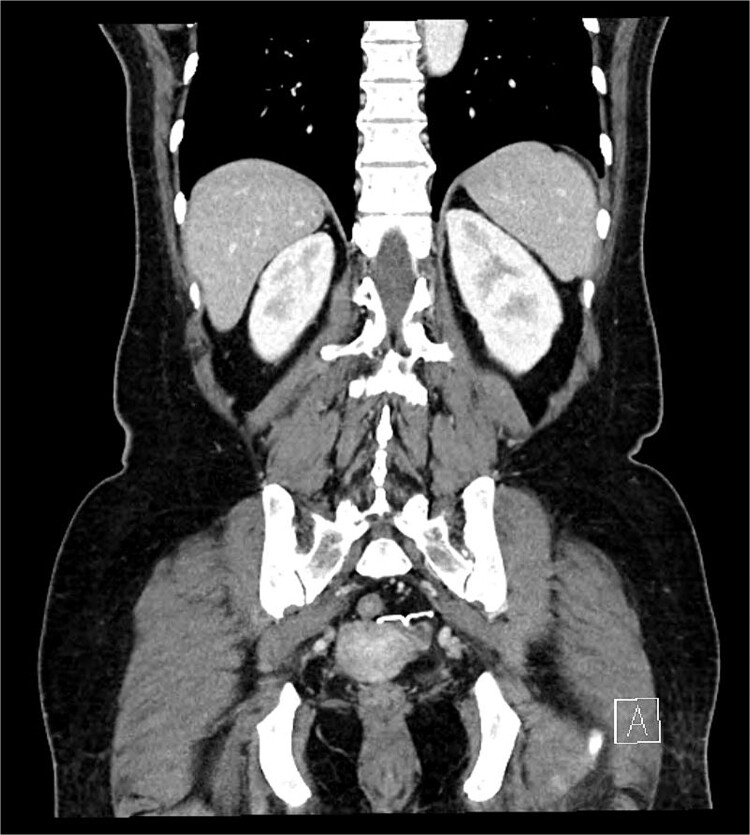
IUD positioning adjacent to the left uterus and extending into the colon, observed on CT.
